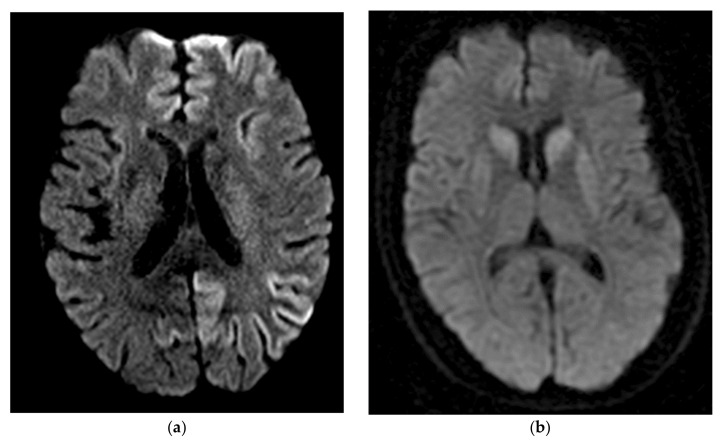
Brain MRI findings in prion disease. (a) Axial DWI imaging showing a widespread cortical restricted diffusion pattern typically found in sCJD MM1; (b) Axial DWI imaging showing restricted diffusion in the bilateral basal ganglia typically seen in sCJD VV2 (ataxic variant).
